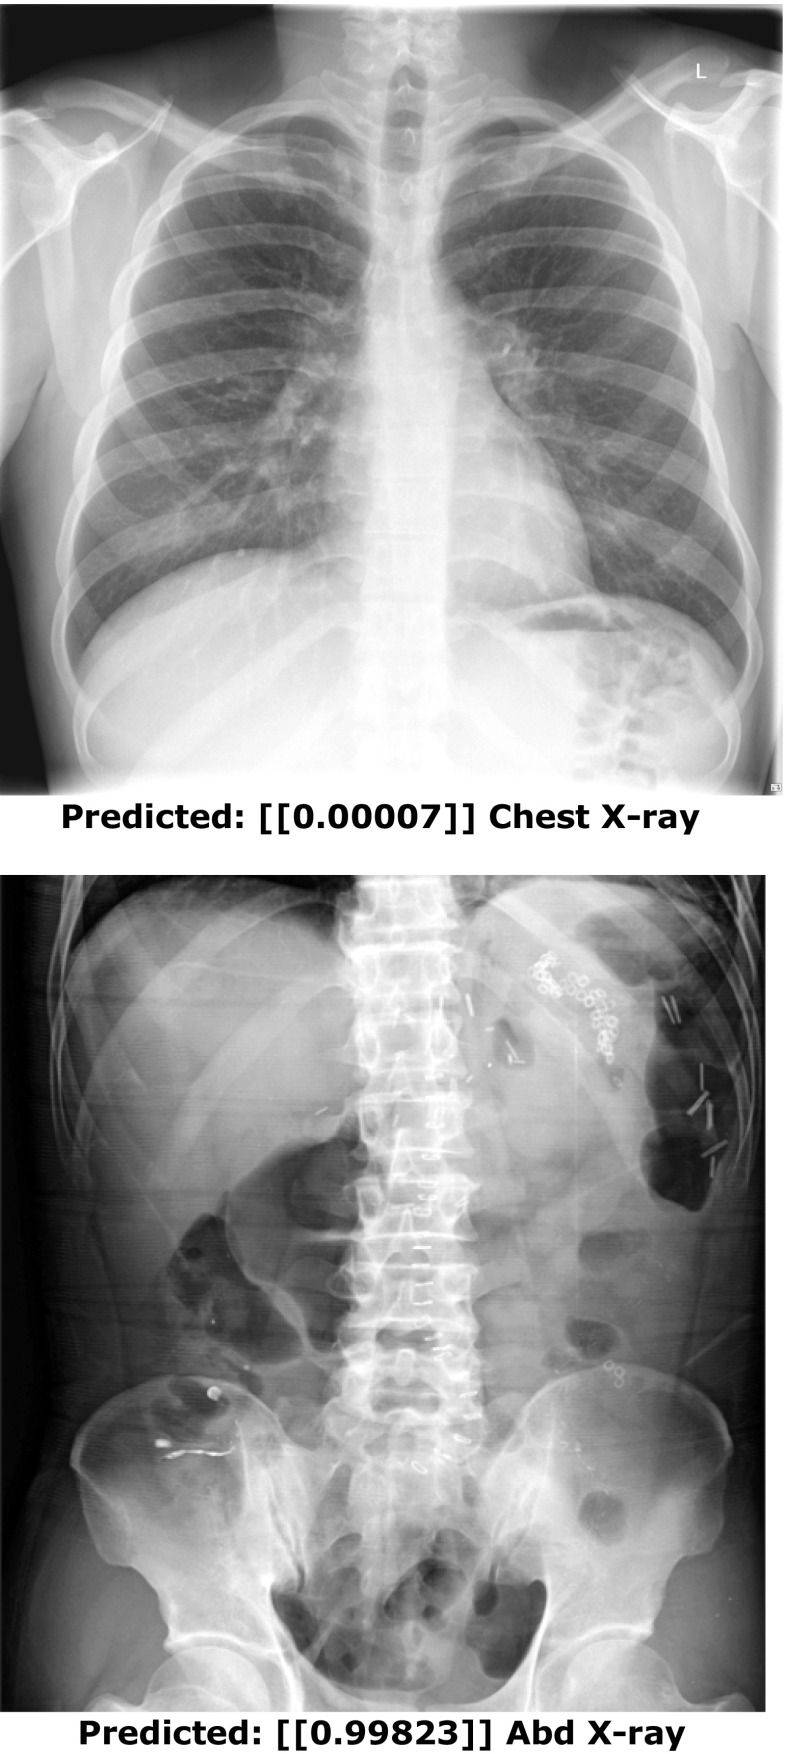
Inference on two test cases. The numbers within the brackets represent the probability of a chest vs. abdominal radiograph (range 0–1). A score close to 0 indicates a high confidence of a chest radiograph, and a score close to 1 indicates a high confidence of an abdominal radiograph
